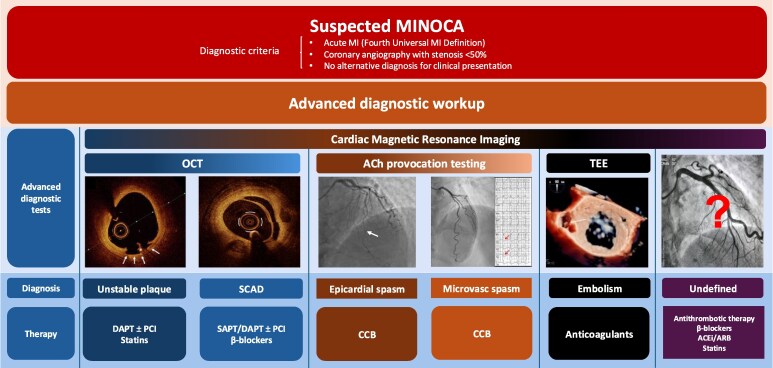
Stratified treatment in MINOCA patients. ACh, acetylcholine; CCB, calcium channel blockers; DAPT, dual antiplatelet therapy; OCT, optical coherence tomography; PCI, percutaneous coronary intervention; SAPT, single antiplatelet therapy; SCAD, spontaneous coronary artery dissection; TEE, transesophageal echocardiography
